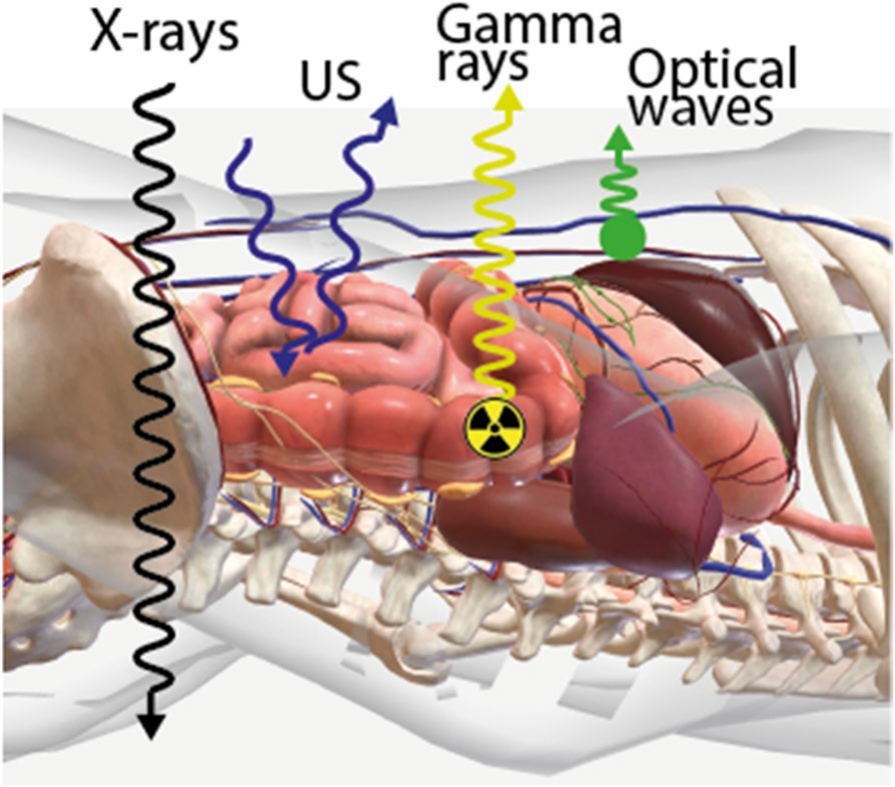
Tissue penetration of different imaging modalities. Each imaging modality uses another type of signal for image reconstruction e.g., X-rays, US, gamma rays and optical waves and therefore suffers from a different signal attenuation resulting in a different penetration capability of the resulting signal
